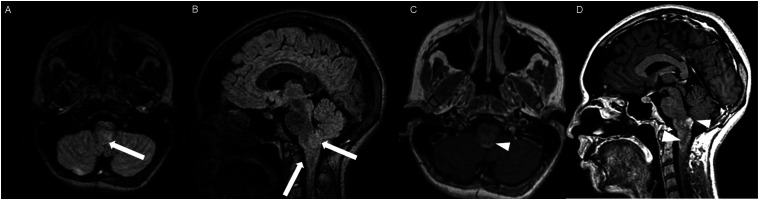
Axial FLAIR (A), sagittal FLAIR (B), axial post-contrast T1 weighted image (C) and sagittal post-contrast T1 weighted image (D) in an NF1 patient with a remote history of resected right cerebellar pilocytic astrocytoma, show an expansible FLAIR hyperintense mass involving the posterior medulla and cervicomedullary junction (A-B, thick white arrows) with heterogeneous striated and nodular enhancement (C-D, arrowhead).
